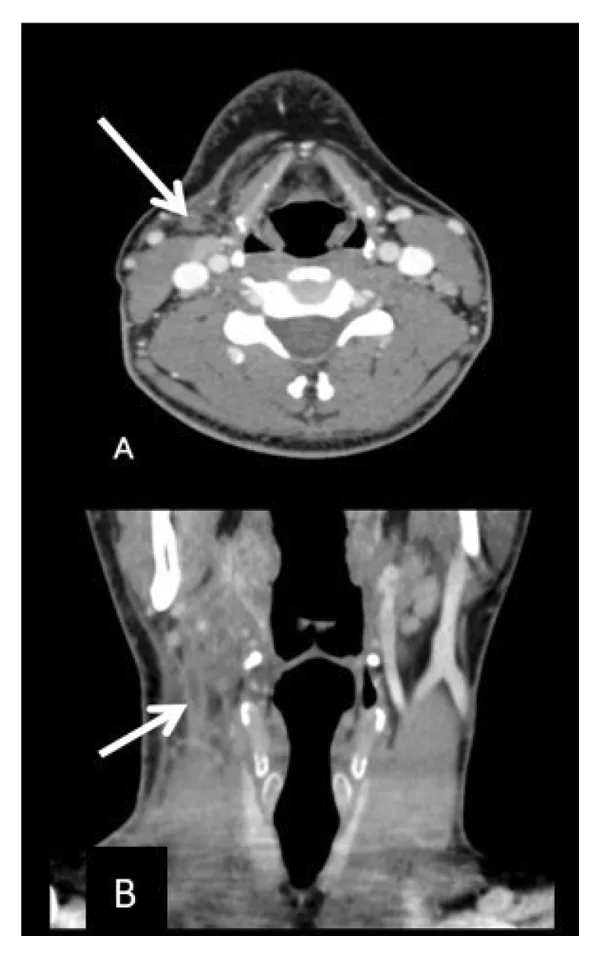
Contrast‐enhanced CT images of the neck showing right submandibular gland swelling and thrombosis of the right external jugular vein in axial (A) and in coronal (B) view.
